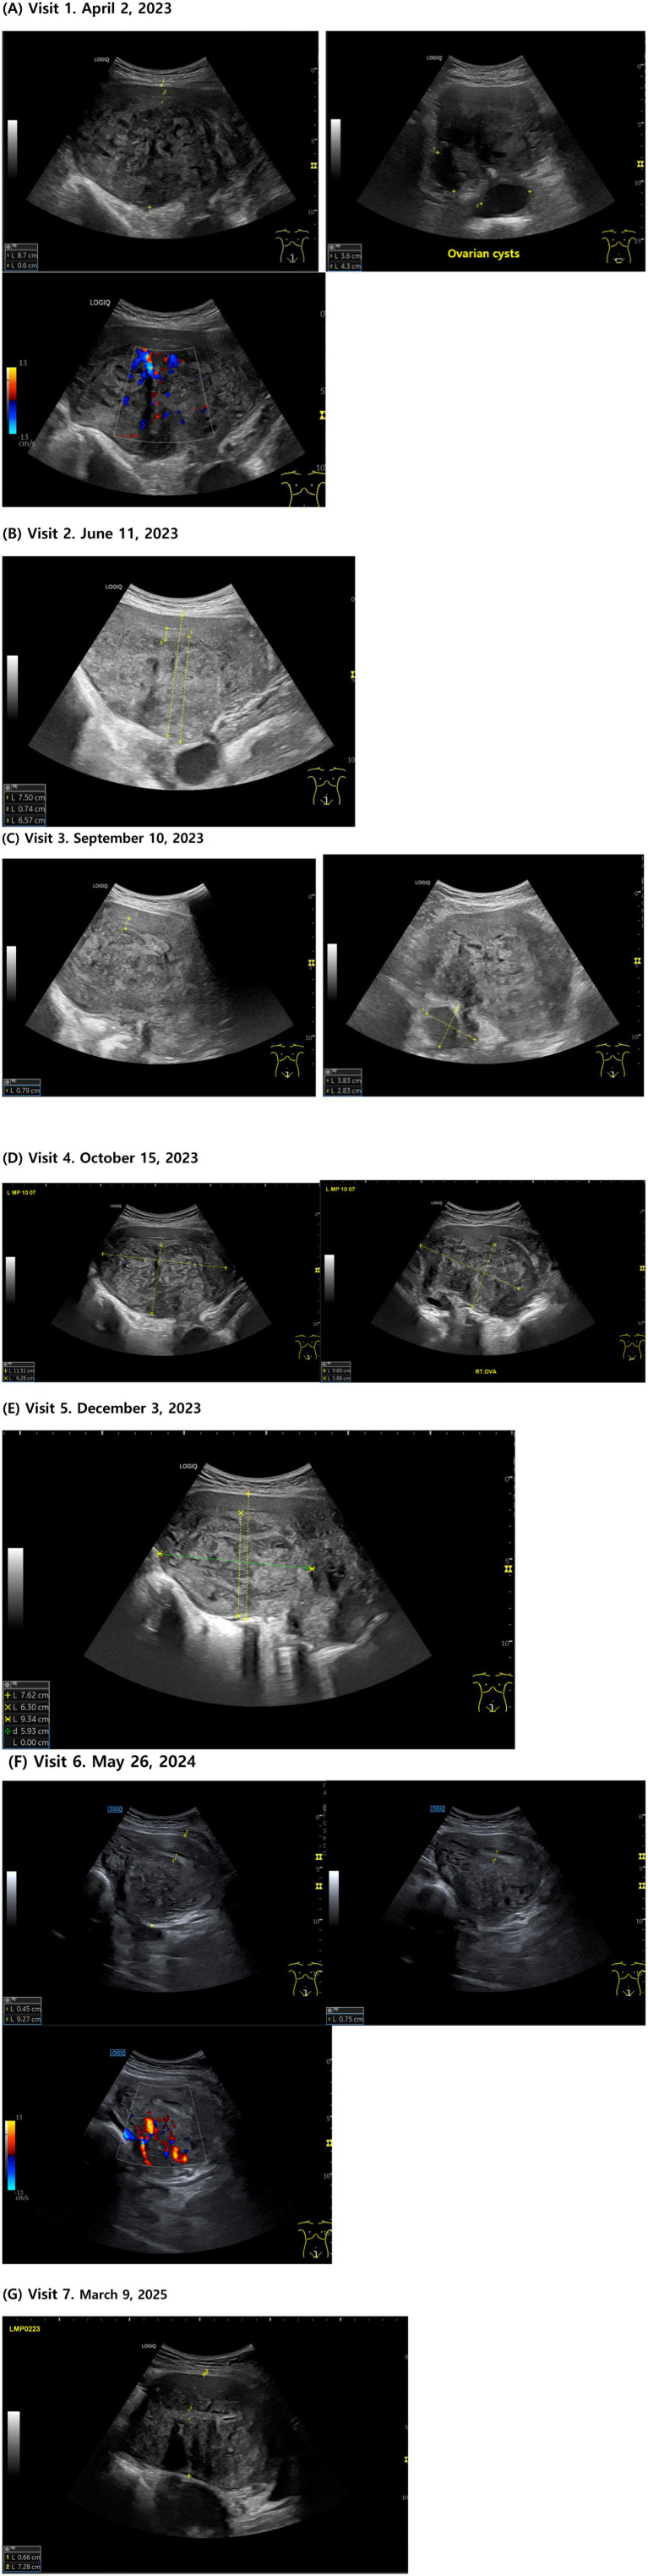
Transabdominal ultrasound images showing adenomyosis. (A) Visit 1. April 2, 2023. (B) Visit 2. June 11, 2023. (C) Visit 3. September 10, 2023. (D) Visit 4. October 15, 2023. (E) Visit 5. December 3, 2023. (F) Visit 6. May 26, 2024. (G) Visit 7. March 9, 2025.
